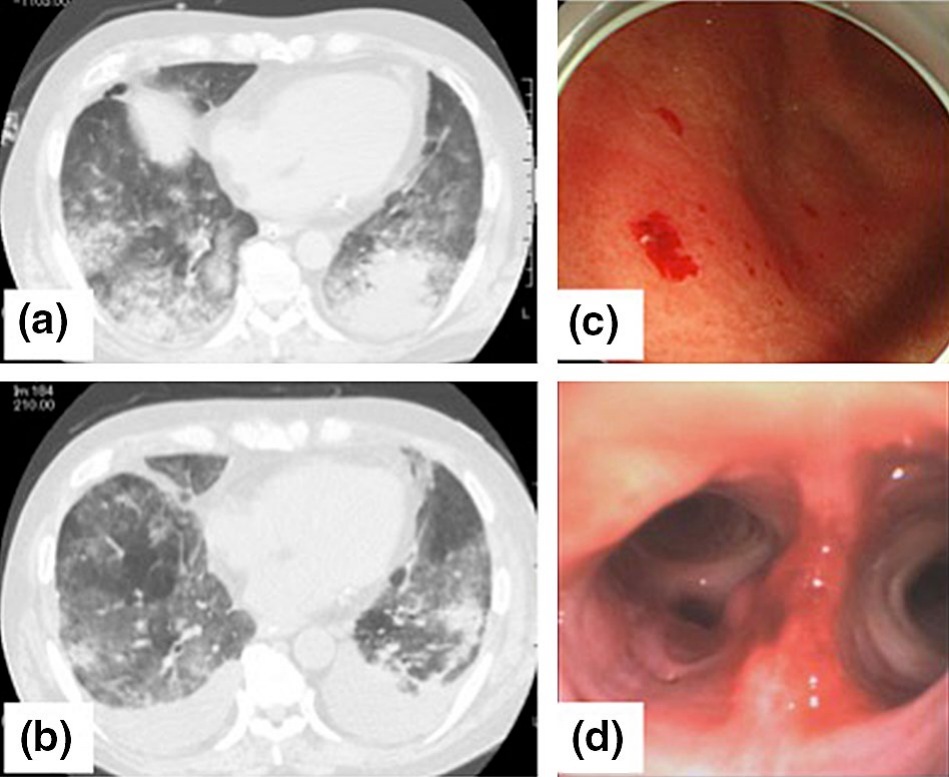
Case 1: a CT scan on arrival and b on the day of the second run. c Gastrointestinal endoscopy shows micro-hemorrhage. d Bronchoscopy shows bloody sputum, blood clots and bleeding from the bronchial wall
